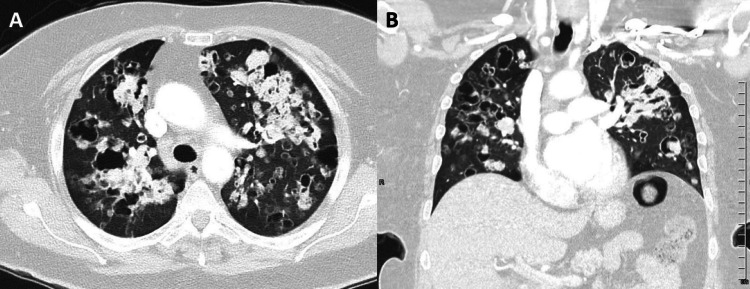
Axial (A) and coronal (B) chest CT images showing disseminated bilateral cystic, solid, and mixed solid/cystic nodules and masses with no pleural involvement
